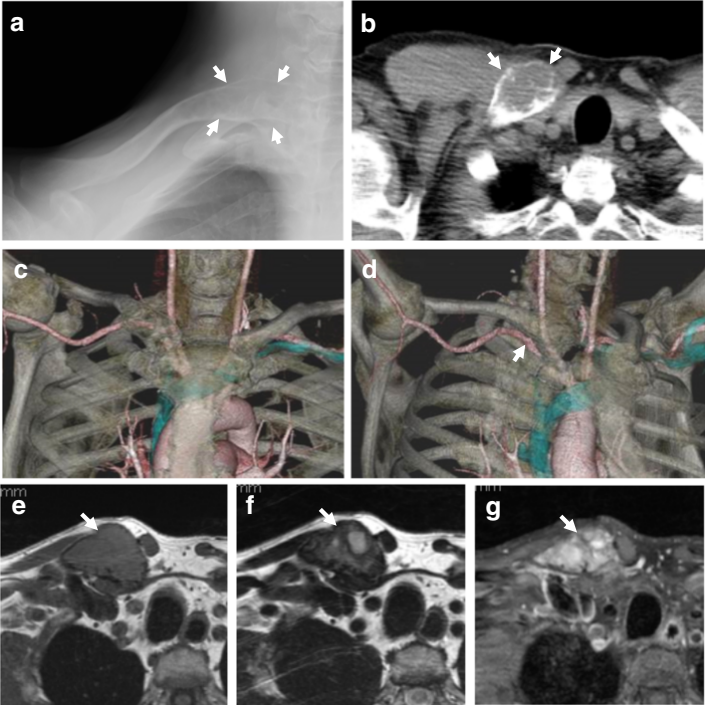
Plain X-ray, computed tomography, and magnetic resonance imaging of the right clavicle. (a) Plain X-ray showed lytic change and ballooning of the proximal end of the right clavicle. (b) Computed tomography (CT) demonstrated an expanded medullary cavity and thinning of the cortex without periosteal reaction. (c, d) 3D-CT angiography images demonstrated proximity of the subclavian artery (*arrow*) and the tumor. The tumor showed (e) isointensity on T1-weighted images and (f) a mixture of low- and high-intensity areas on T2-weighted images. (g) The tumor tissue was significantly enhanced by gadolinium; however, the tumor did not extend to the surrounding soft tissues
